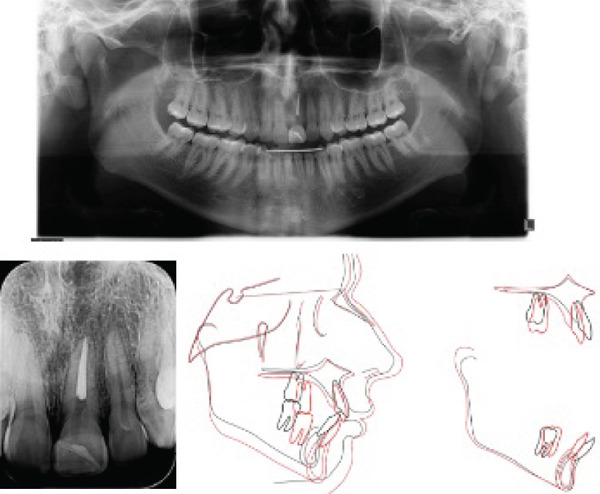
Panoramic and periapical radiographs at the 7 years and 3 months follow‐up and cephalometric superimposition (black, pretreatment; red, posttreatment).
